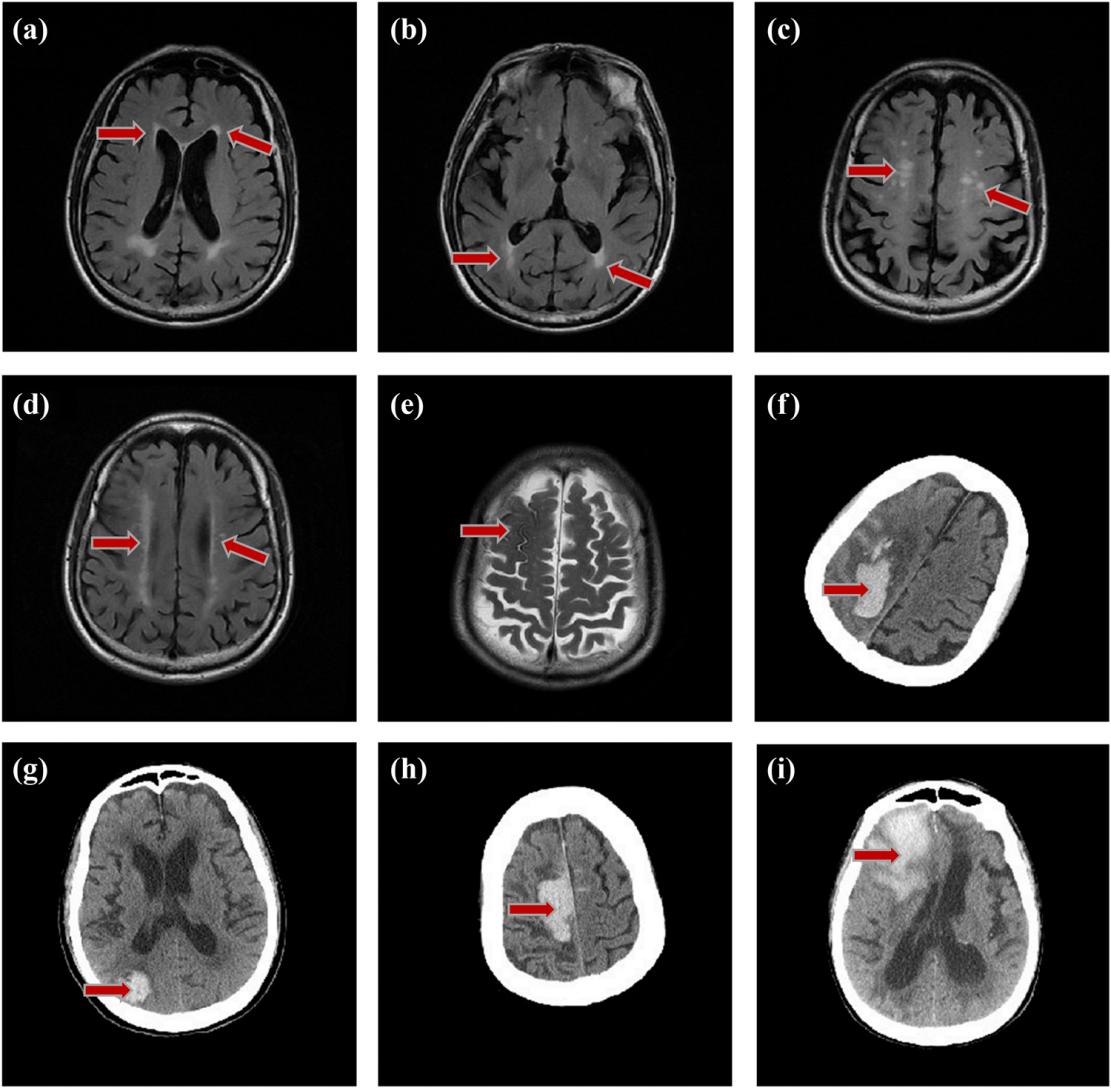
Distribution of early LA and hemorrhages in case 12. An 86-year-old male patient, occipital prominent LA and subcortical patchy distribution of white matter appeared 3 years before the ICH. (a) Score 1 in the frontal lobe white matter (periventricular 1, deep 0, juxtacortical 0). (b) Score 4 in the occipital lobe white matter (periventricular 3, deep 1, juxtacortical 0). (a and b) FO value = −3 occipital prominent LA. (c) Subcortical multiple punctate LA, without clear periventricular white matter damage. (d) The bilateral white matter lesions were asymmetric, with the right white matter lesions severer. (e) T2 shows old convex subarachnoid hemorrhage in August 2009. (f) Shows that ICH occurred in right parietal-occipital lobe in 2012. (g–i) CT shows recurrent ICH occurred in right occipital lobe, parietal lobe, and frontal lobe in 2014, 2016, and 2018, all on the same side of convex subarachnoid hemorrhage.
